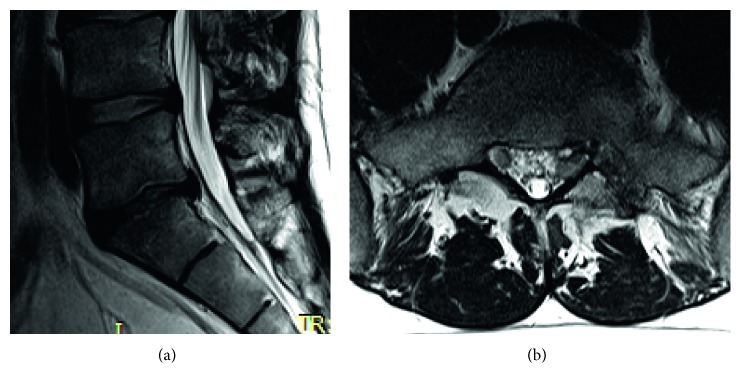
Lumbar MRI of case 1, 4 weeks after surgery.
